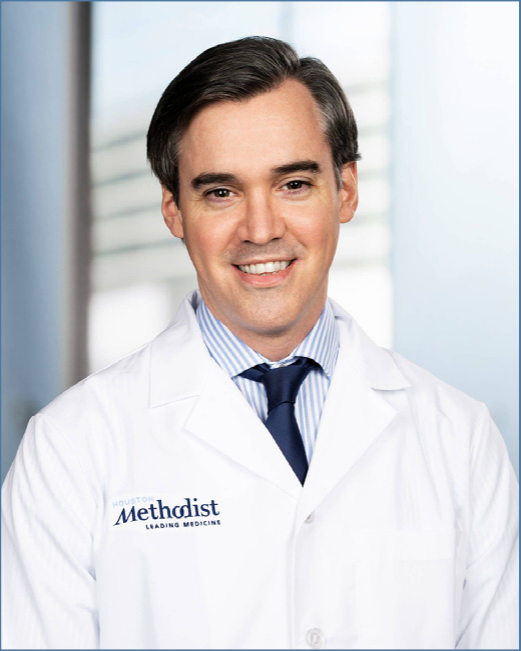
Miguel Cainzos-Achirica, MD, MPH, PhD, joined the Houston Methodist faculty in 2020 after completing his training in cardiology and cardiovascular epidemiology at leading academic institutions in the United States and Europe. He serves as associate director of Preventive Cardiology Research and Education at the Houston Methodist DeBakey Heart & Vascular Center and is an assistant professor of Preventive Cardiology at Cornell Weill and the Houston Methodist Center for Outcomes Research. He is also an adjunct assistant professor of medicine at Johns Hopkins University. With training in cardiology, epidemiology, biostatistics, cardiac imaging, and minority health, Dr. Cainzos-Achirica has research interests in CVD prevention, risk assessment, use of cardiac imaging modalities (particularly computed tomography) to enhance cardiovascular risk stratification, CVD prevention among vulnerable populations (especially people of South Asian ancestry), and advanced epidemiological research methods.
